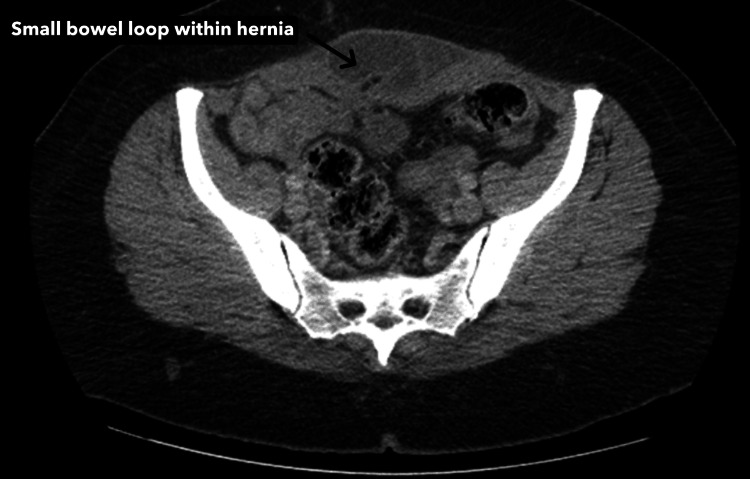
CT scan findings of the small bowel loop within hernia; no contrast seen within the incarcerated loop. The axial slide of the CT with oral contrast shows an incarcerated loop of the small bowel within the hernia. The lack of oral contrast within the incarcerated loop is indicative of obstruction at the site of the hernia.
